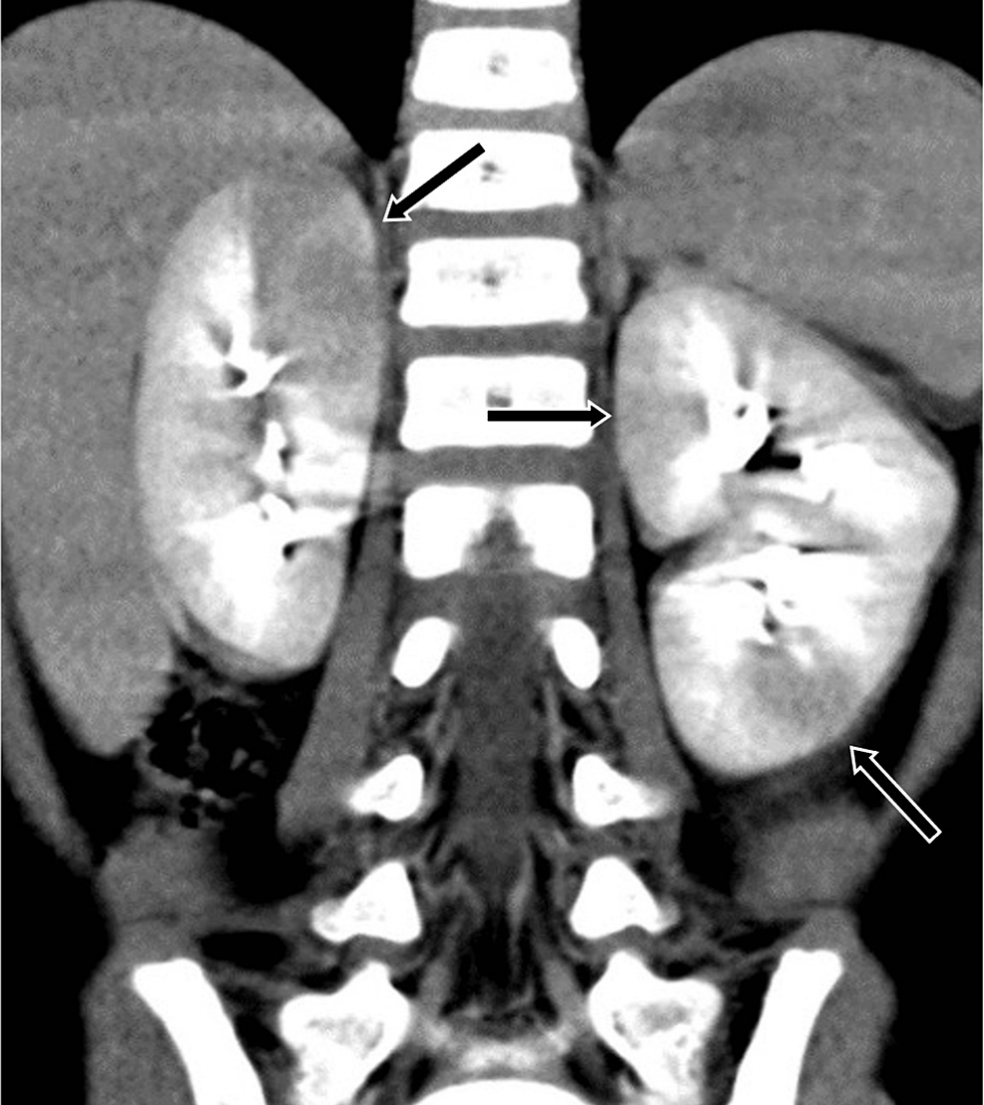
Abdominal contrast-enhanced computed tomography scan on admission Abdominal contrast-enhanced computed tomography (CT) scan on admission revealed multiple, wedge-shaped, low-density areas on both kidneys (arrows).
